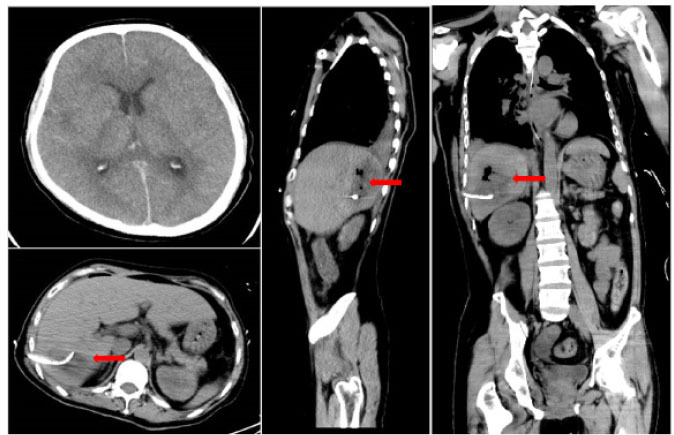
Repeat CT scans on day 2 reveal emerging cerebral edema and subarachnoid hemorrhage, but there is a decrease in hepatic gas and fluid levels. Red arrowhead: after liver abscess puncture drainage, the gas in the abscess cavity had decreased obviously. CT: computed tomography.
